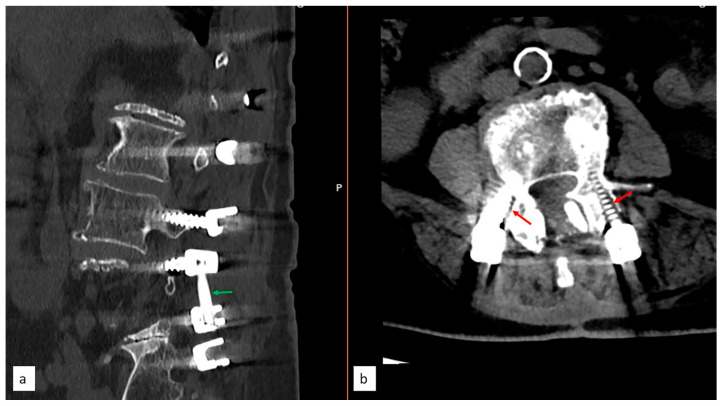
(a). 78-year-old female patient with osteoporotic T-score underwent surgery using a two-stage technique. During the first-stage surgery, decompression at the L4–L5 level was conducted. To prevent the potential development of segmental instability, a temporary peek rod was placed at the level of L4–L5 (Green arrow). (b). After 6 months of osteoporosis treatment, when she presented for the second-stage surgery, a CT scan revealed acceptable osteointegration, but bilateral L4 screws appeared loosened. (Red arrows) These findings supported the theory that surgeries performed in a single stage may cause overload on the screws and lead to loosening.
